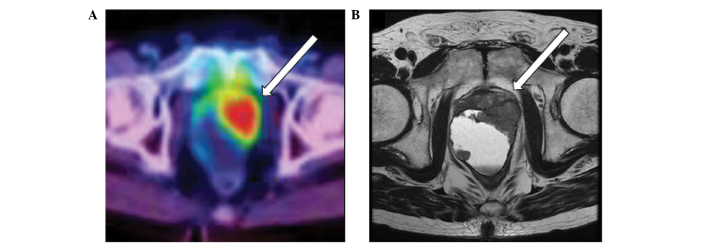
(A) [^18^F]-fluoro-deoxy-2-glucose positron emission tomography/computed tomography showing strong accumulation in the pelvis (arrow), and (B) pelvic magnetic resonance imaging showing a cystic lesion (arrow) behind the prostate gland. The wall of the cyst is partially thickened, and the surface of the thickened wall is irregular.
